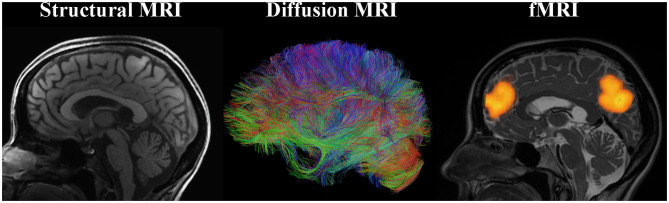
Main MRI modalities for measuring training-induced plasticity.
